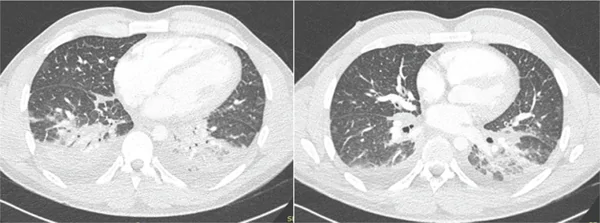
Chest computed tomography pulmonary angiography excluding pulmonary embolism and demonstrating bilateral bibasilar consolidations with areas of alveolar filling predominantly in the lower lobes, associated inflammatory airway changes, moderate bilateral pleural effusions, and features of interstitial pulmonary edema.
